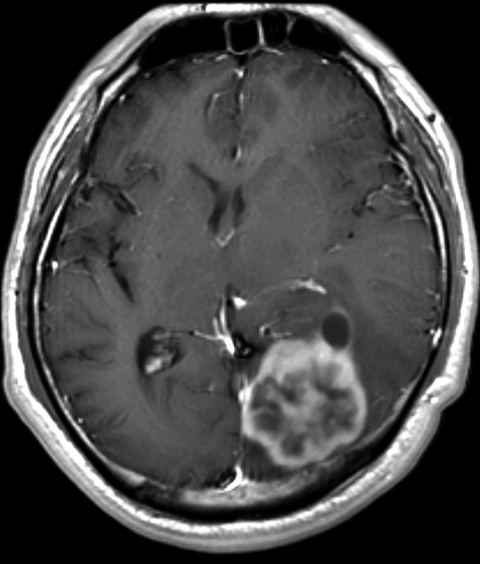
Postcontrast T1-weighted magnetic resononance image of the brain of a 44-year-old man with cerebral cryptococomma in Japan, 2007, showing a rim-enhancing lobulated mass (lower right) with surrounding edema in the left occipital lobe.
